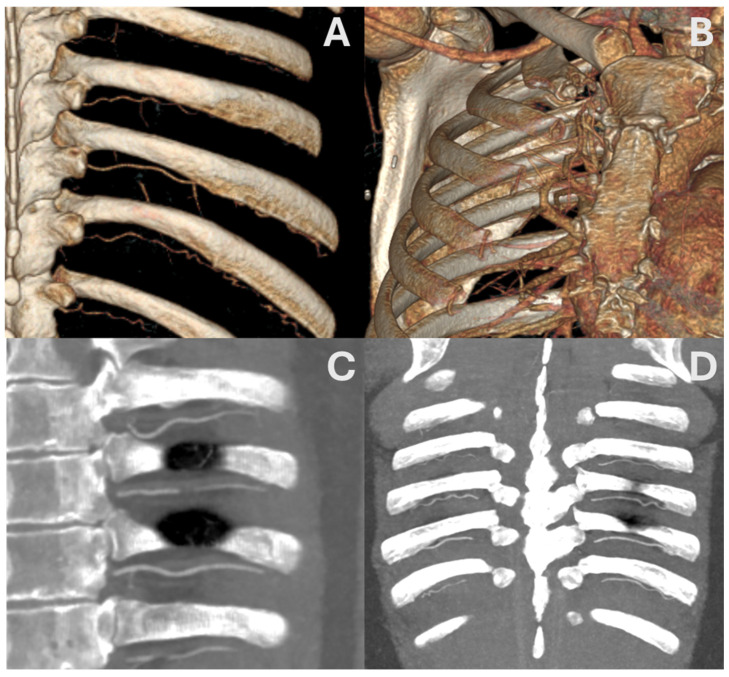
Contrast-enhanced CT acquired at the arterial phase. Volume rendering reconstruction showing left (A) and right (B) posterior intercostal arteries. Oblique reformatted image with maximum-intensity-projection reconstruction showing left posterior intercostal arteries (C). Coronal reformatted image with maximum-intensity-projection reconstruction showing both right and left posterior intercostal arteries (D).
